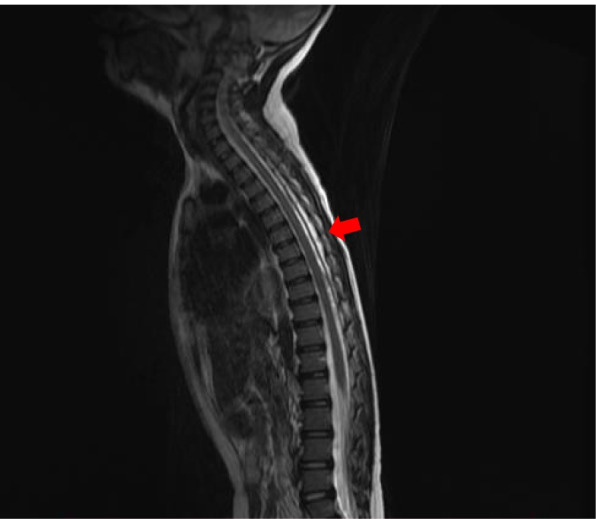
Sagittal T2-weighted whole spine magnetic resonance imagings (MRIs) of the patient indicating syringomyelia at T2–T7 level (arrow)
